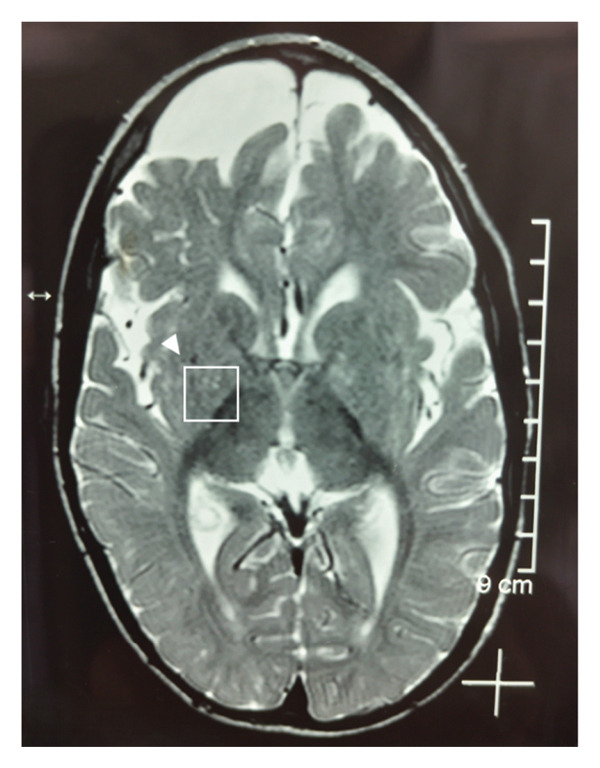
Case 1: Axial T2‐weighted MRI image at 5 years, 11 months of age. White box and arrowhead indicate region of interest in the lentiform nucleus. Confluent and diffuse hypomyelination was confirmed.
